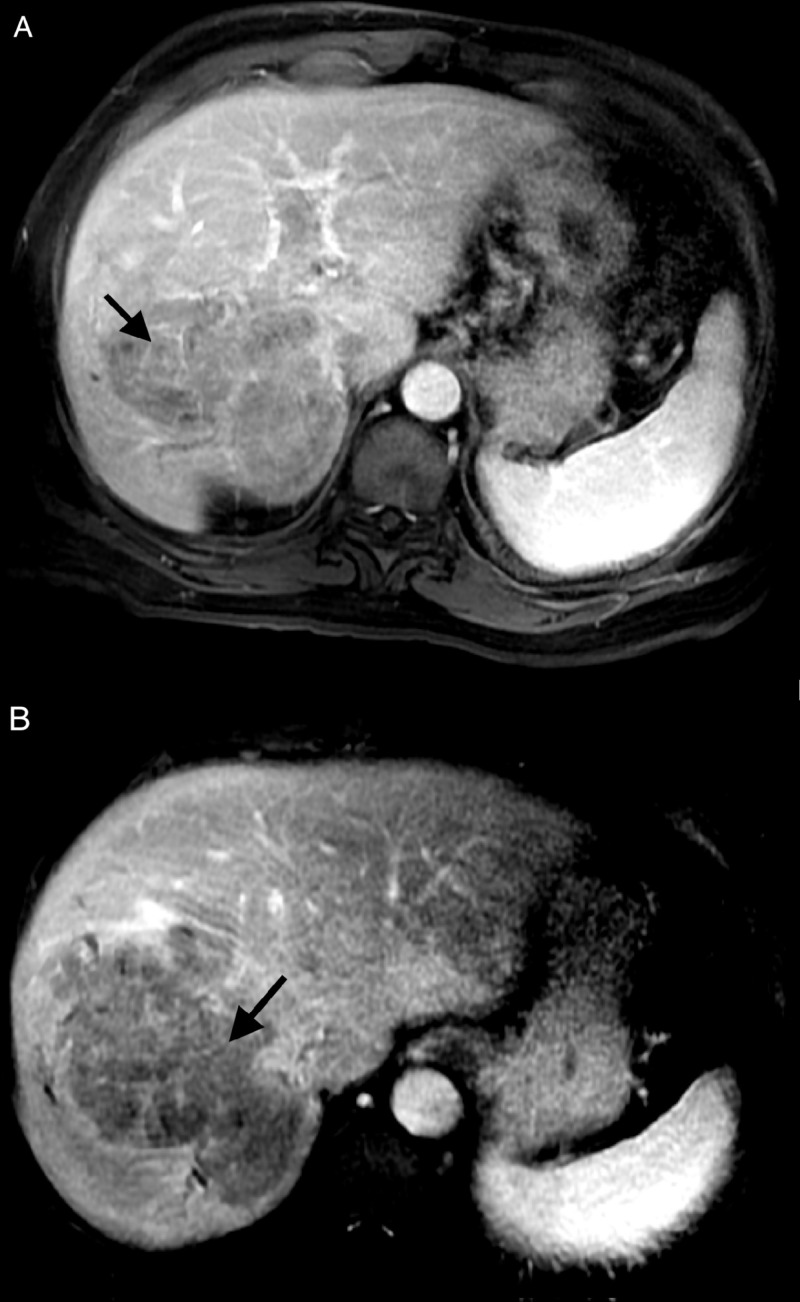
June 2017 MRI with IV contrast A) Arterial phase 20-second LAVA sequence; B) venous phase one-minute LAVA sequence MRI: magnetic resonance imaging; IV: intravenous; LAVA: liver acquisition with volume acquisition
